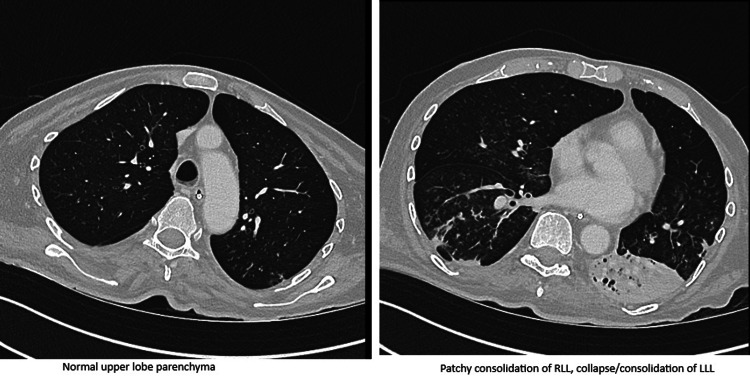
CT scan of the chest shows patchy consolidation in the right lower lobe and collapse/consolidation in the left lower lobe. Otherwise, the lung parenchyma is normal, with no interstitial lung disease (ILD) changes.
